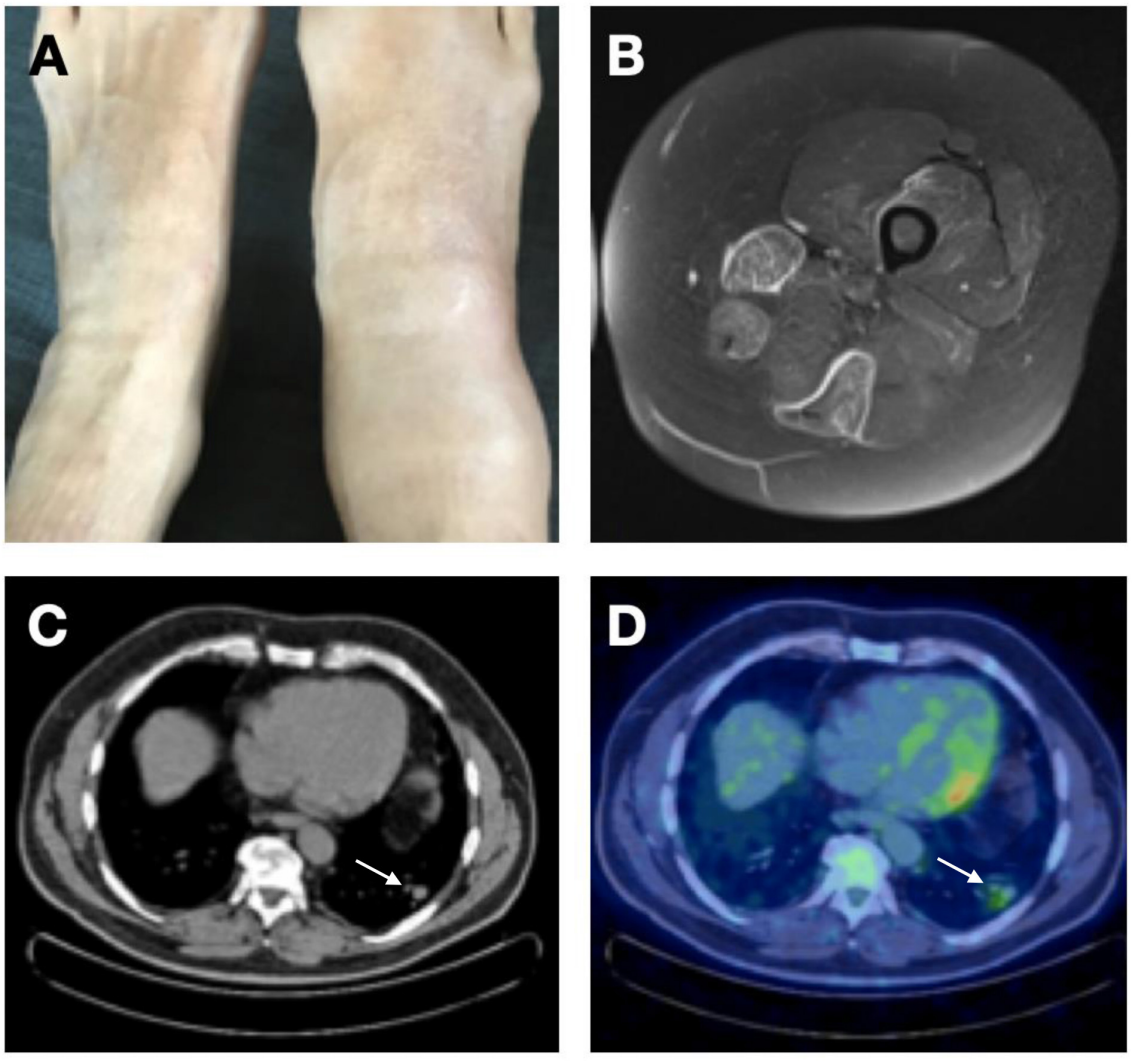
Non-cutaneous complications of CD3^−^CD4^+^ T cell associated L-HES. (A) P3, arthritis and synovitis right ankle. (B) P11, fasciitis—MRI showing gadolinium enhancement of the fascia and underlying sartorius and gracilis muscles of right leg. (C,D) P13, pulmonary involvement—Thoracic CT (C) scan showing nodule in left lower lobe (see white arrow), with mild to moderate hypermetabolic activity shown by FDG-PET scan (D).
